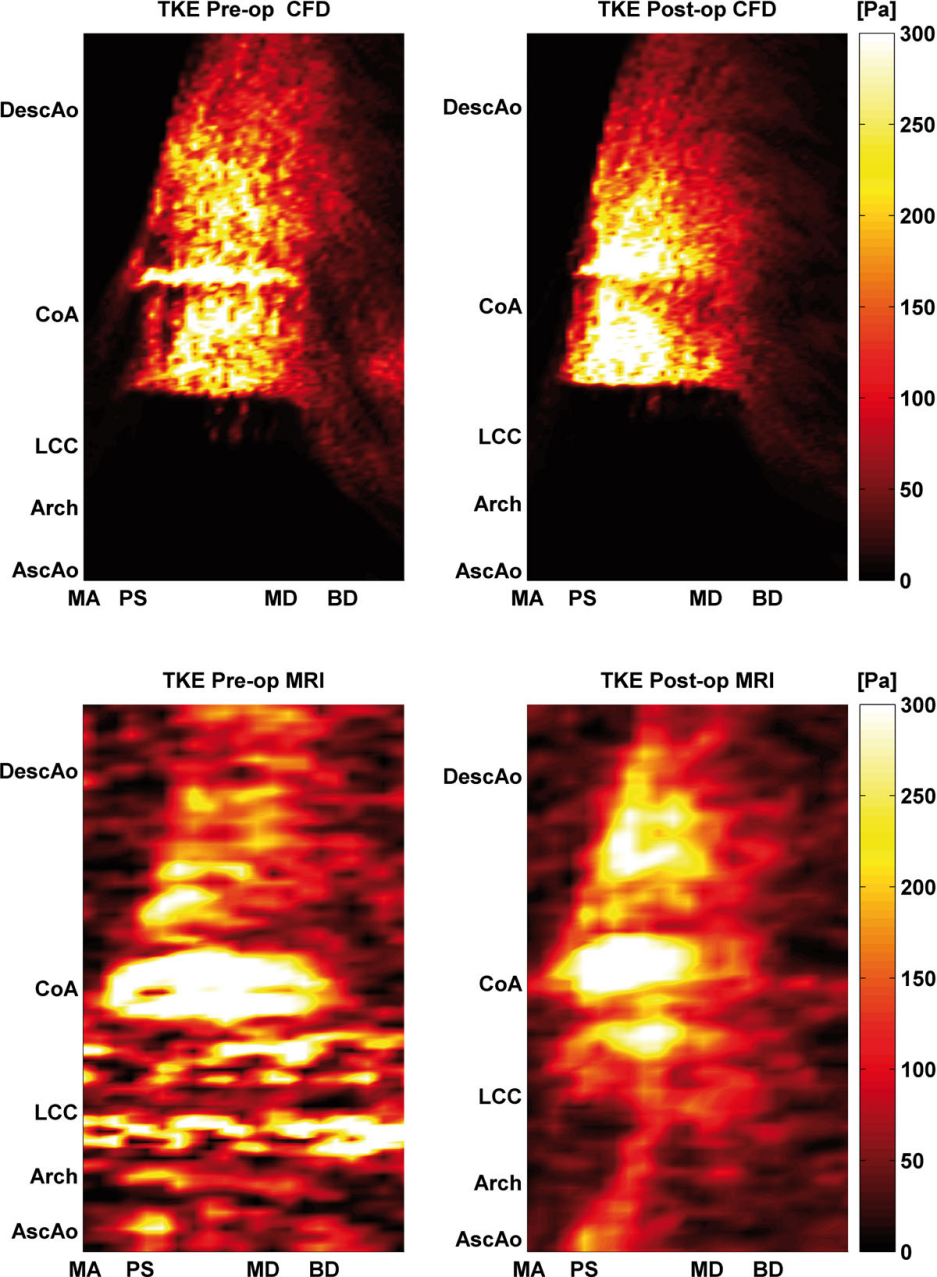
TKE along the centerline as a function of time in the pre- and post-intervention cases; comparison between CFD (top row) and MRI (lower row). X-axis labels: MA: maximum acceleration, PS: peak systole, MD: maximum deceleration, BD: beginning of diastole. Y-axis locations are AscAo: ascending aorta, Arch: aortic arch, LCC: left common carotid artery, CoA: coarctation of the aorta, and DescAo: descending aorta.
